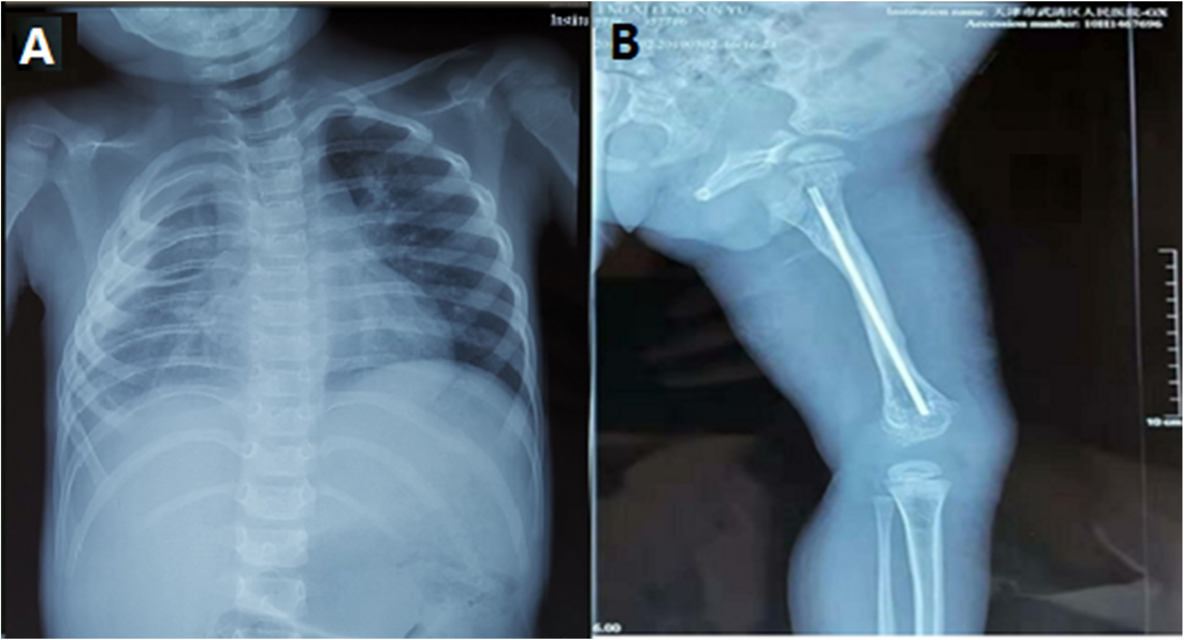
(a) X-ray showing a little pleural effusion in the right thoracic cavity. (b) X-ray of the left femur showing that the bone is remodelling well
